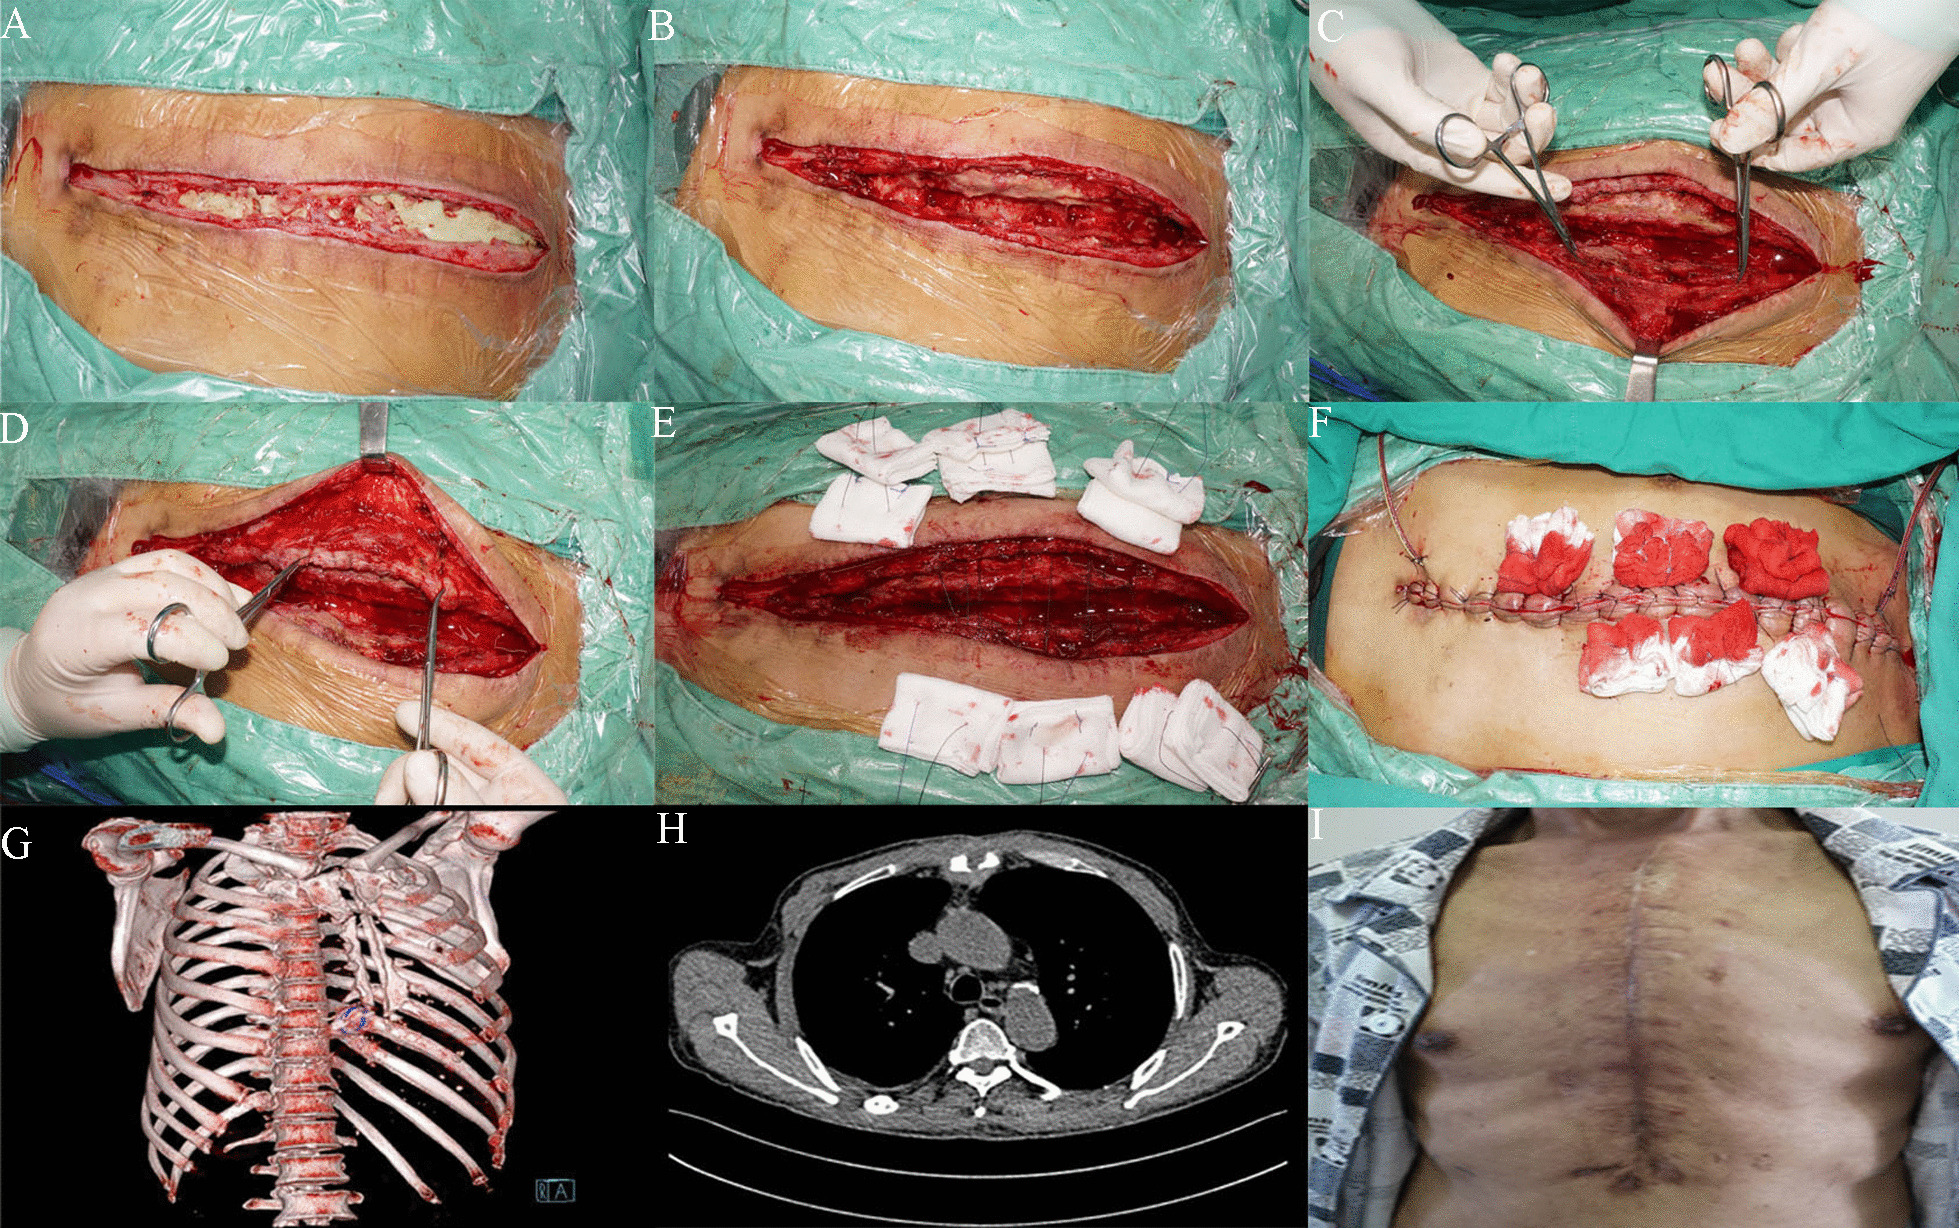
II-stage surgical procedure of the DSWI. a ALBC covering the surface of sternal; b The wound after removing the retained ALBC and complete debridement; c Mobilization of left pectoralis major muscle flap; d Mobilization of right pectoralis major muscle flap; e The subcutaneous tissue and pectoralis major muscle flap were intermittently sutured by methods of relieving tension and no residual foreign bodies; f Drain tubes were placed: one under the muscle flap and the other under the subcutaneous layer; g Three-dimensional rib reconstruction revealed sternal dehiscence; h CT image of 3 months after final surgical reconstruction; i Healed sternal wound at the 3-month postoperative visit
